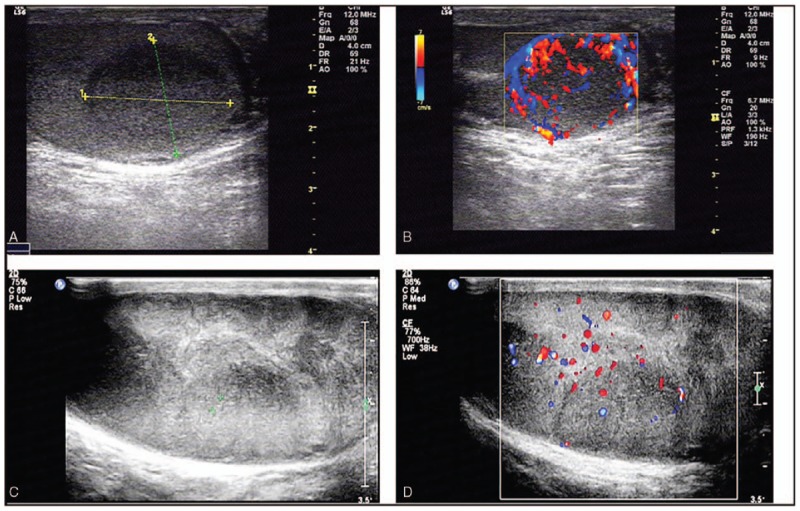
Ultrasound results: a-b, Limited type: The size of the right testicle was normal, 40 × 30 × 26 mm. Hypoechoic nodules with clear boundaries and regular morphology were present in the testicular parenchyma (a). CDFI showed abundant blood flow signal; Alder blood flow grade: III (patient 8) (b). c-d. Diffuse type: The volume of the left testicle was increased, 51 × 41 × 26 mm. The shape was regular, and the diffuse echo of the parenchyma was decreased. The distribution was not uniform, and there was no echo of normal testicular tissue (c). CDFI showed abundant blood flow signal; Alder blood flow grade: III (patient 2) (d).
